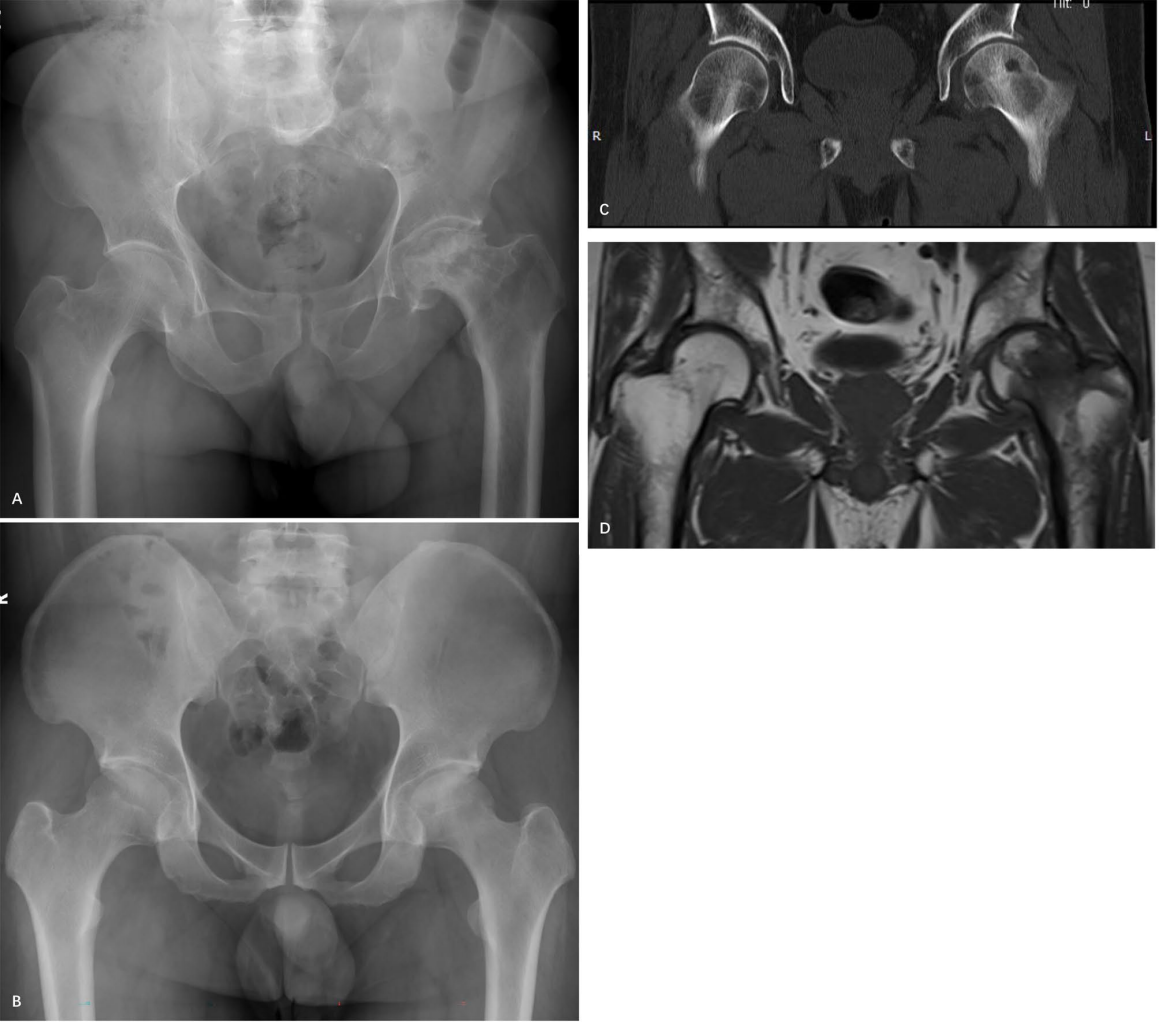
A 60-year-old male patient with an ONFH of his left hip. The patient had undergone CD surgery 2 years previously. (A) The radiograph showed flattening of his left femoral head. (B) Preoperative X-rays. (C) Preoperative CT. (D) Preoperative MRI.
